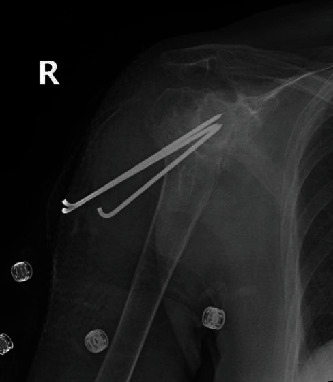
Postoperative plain radiographs of the right shoulder showing percutaneous pinning of the proximal humerus fracture using three k-wires.
